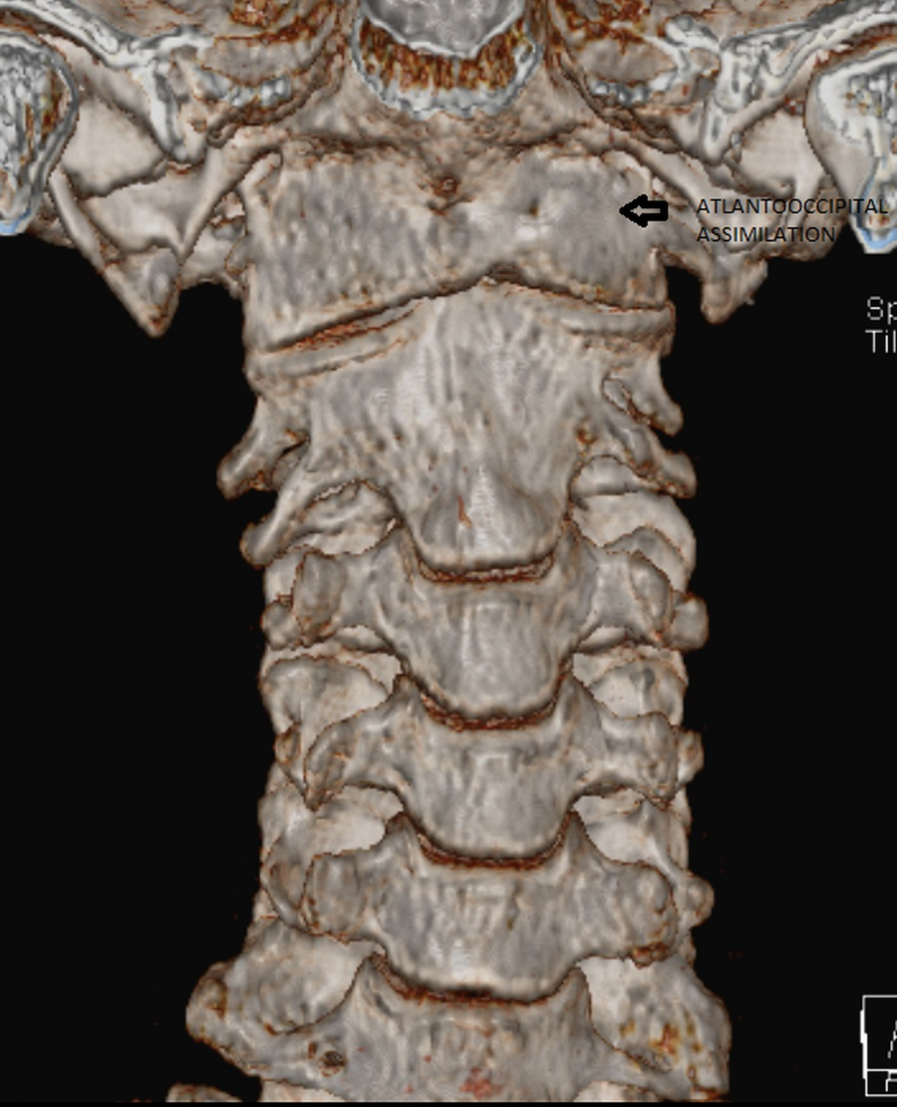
3D computed tomography scan showing complete atlantooccipital assimilation (arrow)
